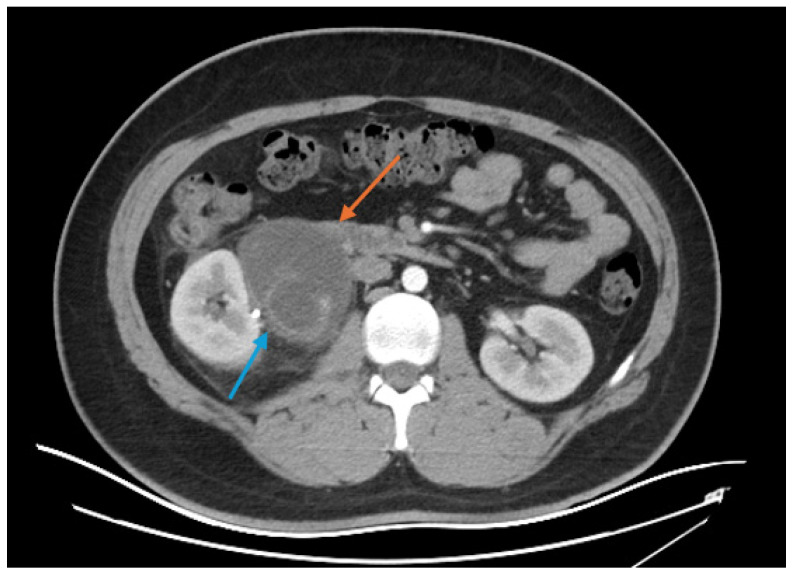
Cross-sectional CT scan of the abdomen before the 1st surgery: abnormal fluid collection around the kidneys (orange arrow); rupture of the renal pelvis (blue arrow).
